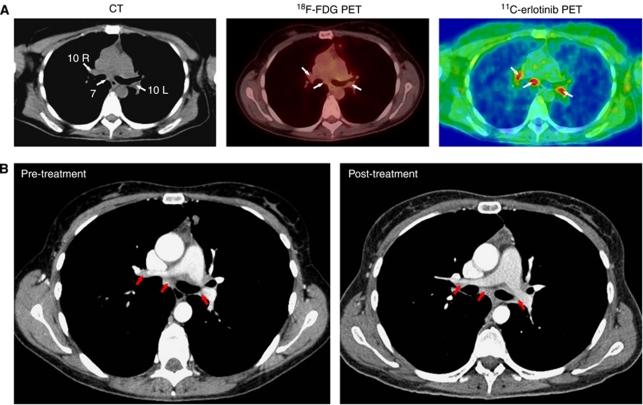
^11^C-erlotinib accumulation in lymph nodes that were negative on ^18^F-FDG PET/CT in a 42-year-old patient (no. 6). (A) Left: transaxial slices of contrast-enhanced CT; middle: ^18^F-FDG PET/low-dose CT; right: ^11^C-erlotinib PET/low-dose CT. CT (left figure) showed an enlarged lymph node (>10 mm) at position 7 (arrow) and non-enlarged lymph nodes (<10 mm) at positions 10R and 10L (arrows). None of these lymph nodes were visualized by ^18^F-FDG PET/CT (arrows, middle figure), whereas both enlarged and non-enlarged lymph nodes were visualized by ^11^C-erlotinib PET/CT (arrows, right figure). The ratio between ^11^C-erlotinib average radioactivity concentrations in the lymph nodes and that in surrounding lung tissue was 2. (B) Comparison of pre-treatment and 1-year post-treatment CT scans showed no significant change in the size of any of these lymph nodes.
